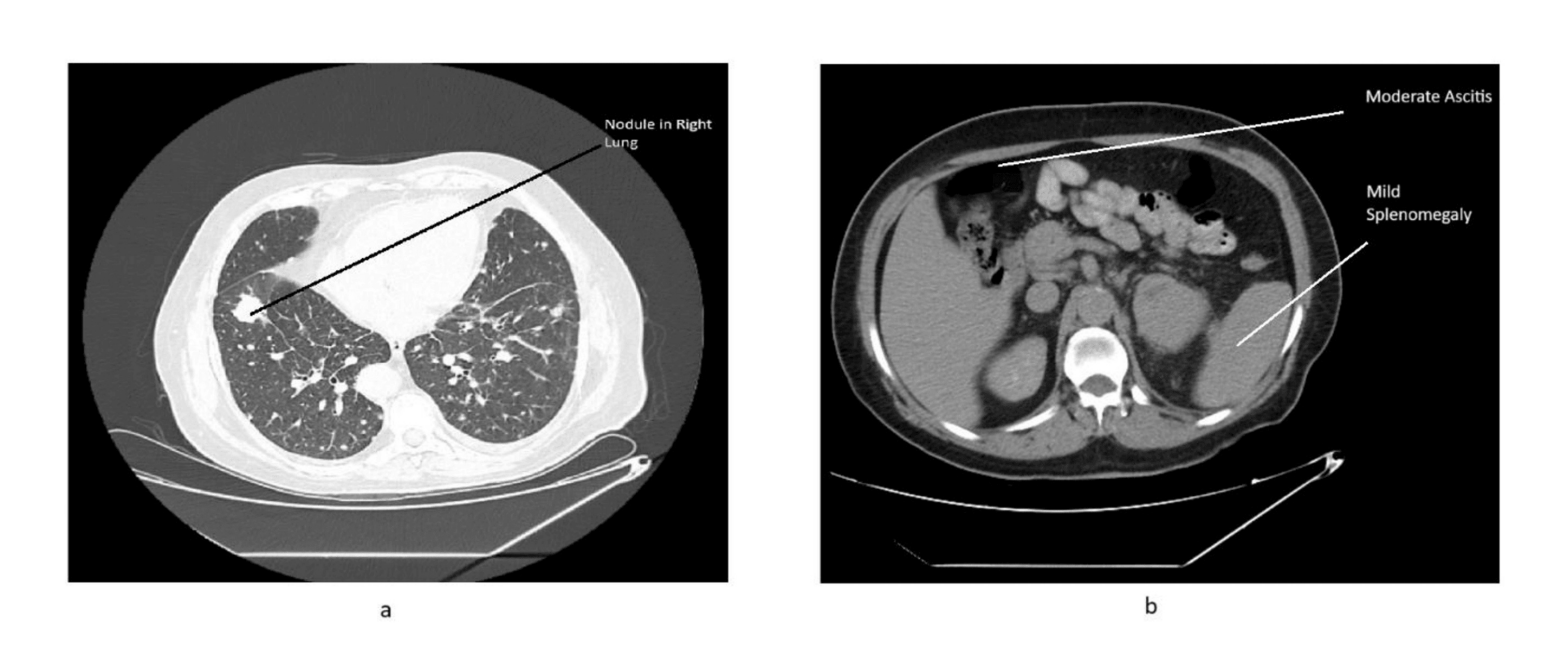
a) CT thorax and b) CT abdomen A CT scan of the abdomen revealed moderate ascites and mild splenomegaly with the spleen measuring 12.4 cm. A CT thorax revealed bilateral lung nodules, with the most prominent visible white rounded lesion in the right lung. CT, computed tomography
